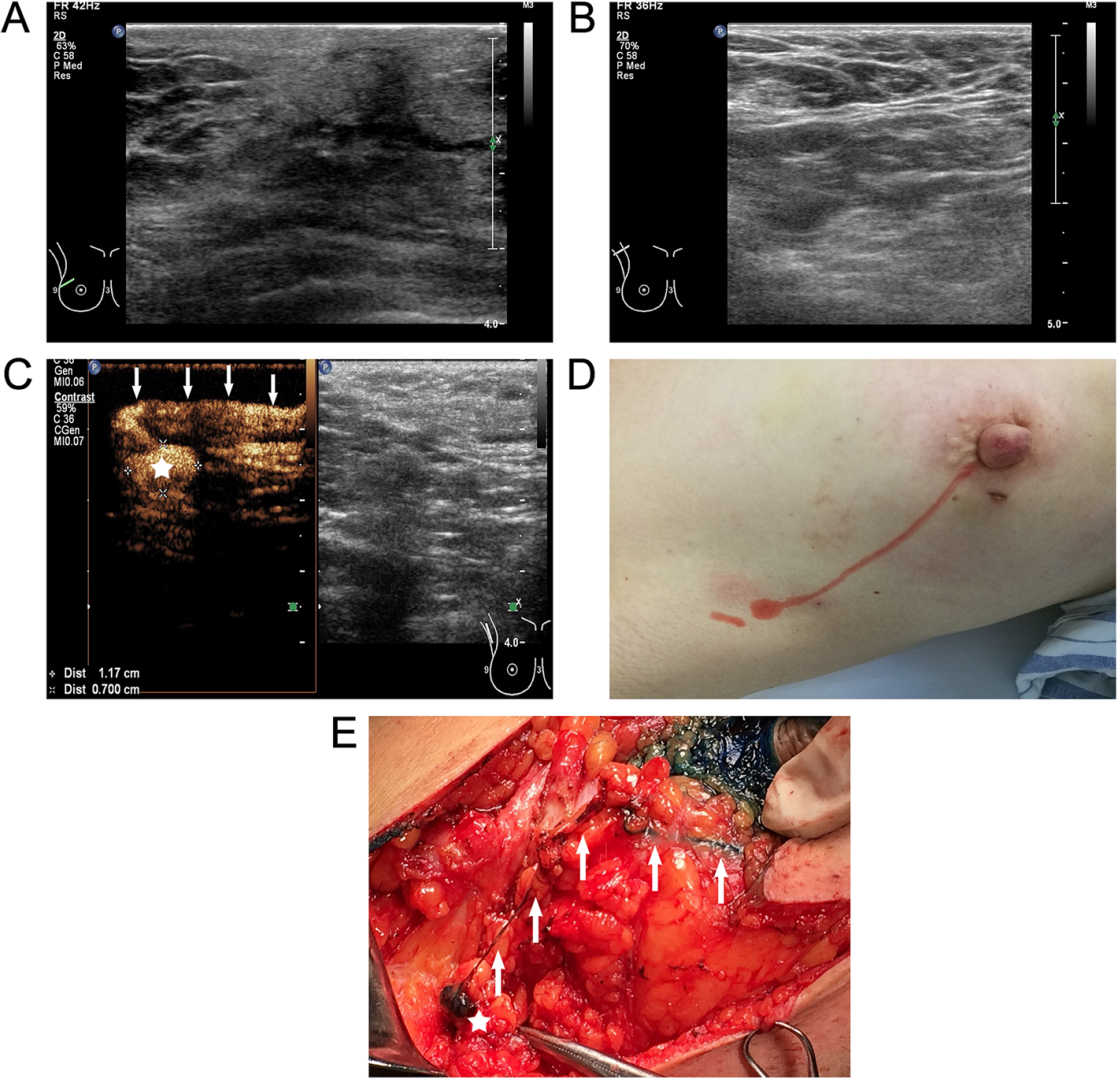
Case1, 54 year-old woman, Ductal carcinoma in situ. a B-Mode Ultrasonography of right external upper quadrant ductal carcinoma in situ; b B-Mode Ultrasonography of the right axillary; c traced the enhanced SLN (asterisk) along the enhanced lymphatic vessel (arrows) after injecting the UCA; dThe body surface projection of enhanced lymphatic vessel and lymph node; eTraced the dyed SLN (asterisk) along the dyed lymphatic vessel (arrows) after injecting the MB before surgery, the lymphatic drainage pattern traced by CEUS was consistent with MB
